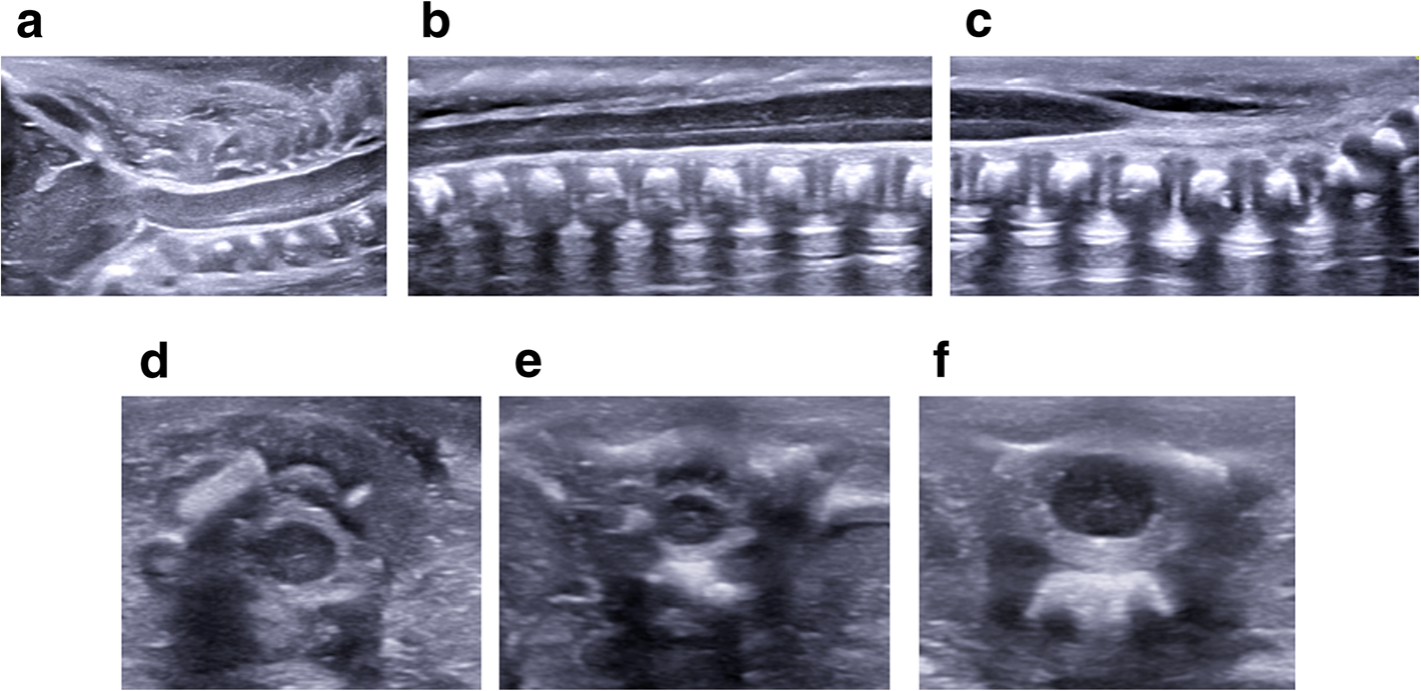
Sagittal postmortem ultrasound images of a normal cervical spine (a), thoracolumbar spine (b) and lumbosacral spine (c) in a 21-week gestational-aged foetus, obtained 12 days after death. Corresponding transverse views of the cervical (d), thoracic (e) and lumbar (f) spinal cord are also shown from a routine normal postmortem ultrasound examination
